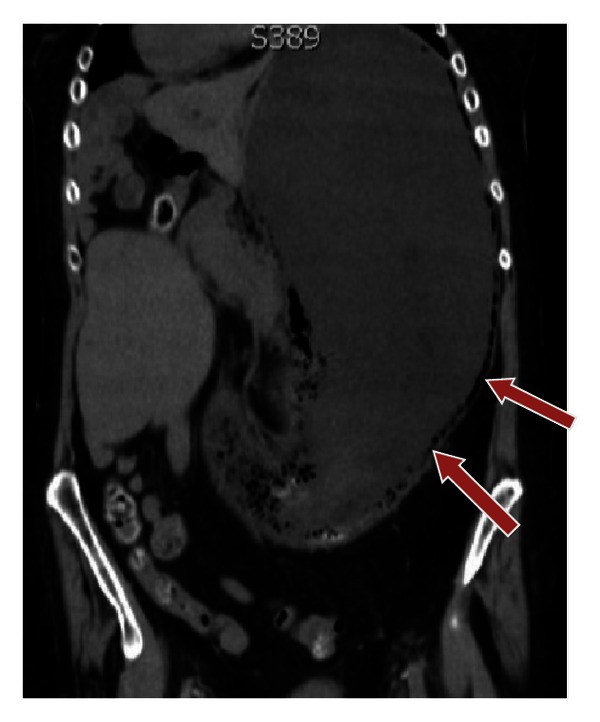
Gastric emphysema on CT scan, coronal reformat (arrows).
